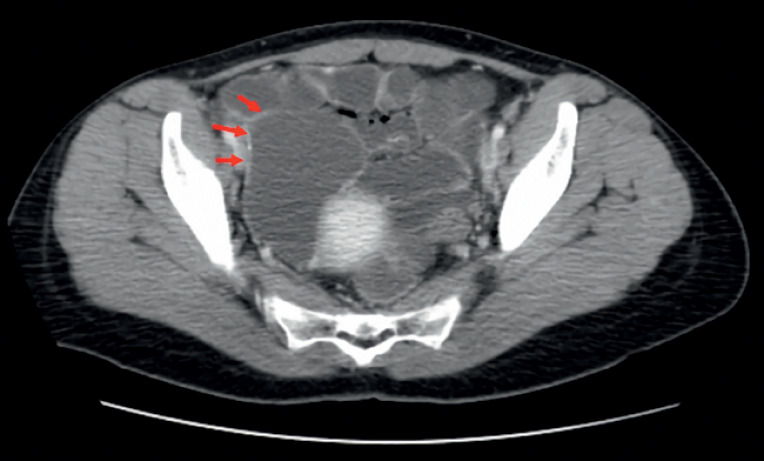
Small bowel CT enhancement, suggesting an irregular cystic lesion on the right side of the uterus, with no significant enhancement observed on enhanced scanning.
